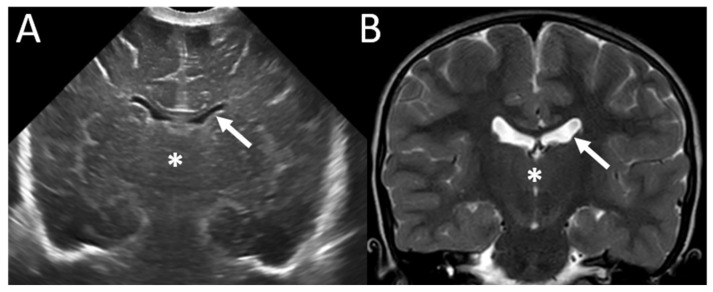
Postnatal transfontanellar ultrasounds (A) and brain MR ((B) coronal T2 weighted images) images, at 9 days and 4 years, respectively, showed the presence of an atretic third ventricle with aspects of partial fusion of the thalami on the midline (*) without ventriculomegaly (arrows).
